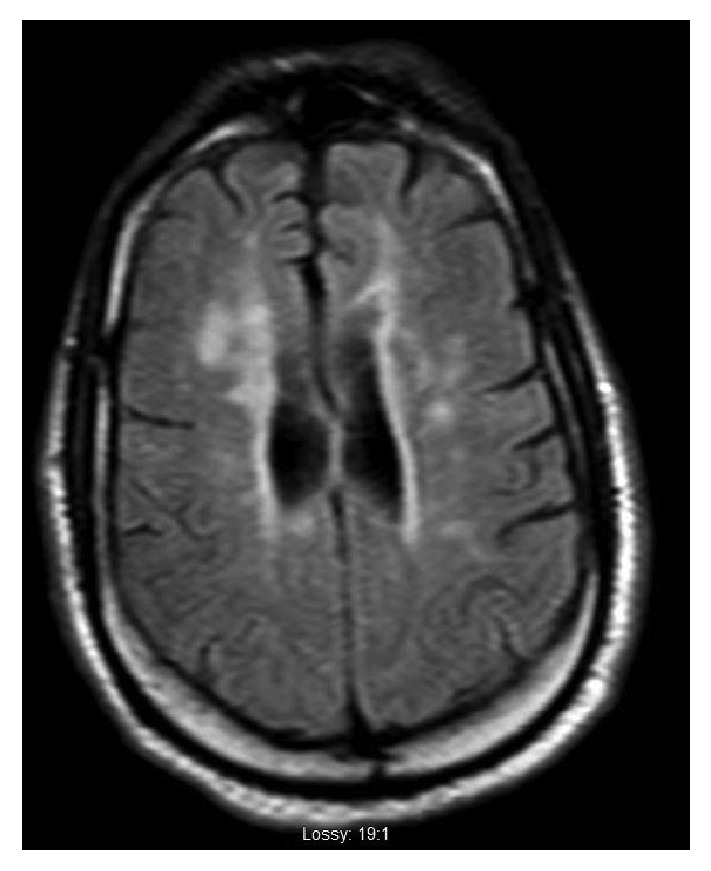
Abnormal hyperintense periventricular white matter lesions on MRI brain T2 FLAIR sequencing.
